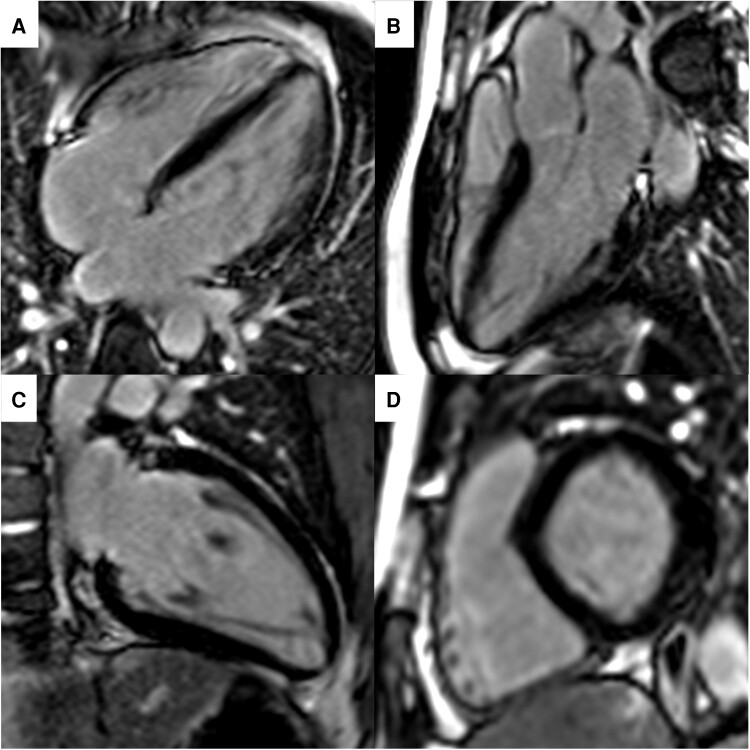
Late gadolinium enhanced cardiac MRI images acquired four months following discharge from hospital. Four-chamber (*A*), three-chamber (*B*), two-chamber (*C*), and basal short axis (*D*) views show subtle patchy enhancement in the basal-to-mid lateral wall. Short axis slice in the basal ventricle (h) also demonstrates subtle enhancement in the basal lateral wall. The lateral wall is now of normal thickness.
